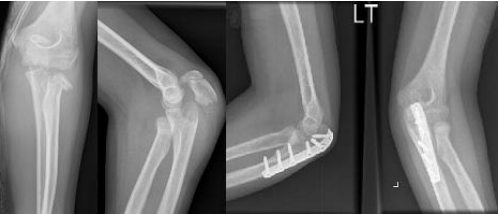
Radiographs showing a Monteggia type I equivalent elbow injury before and after internal fixation.
